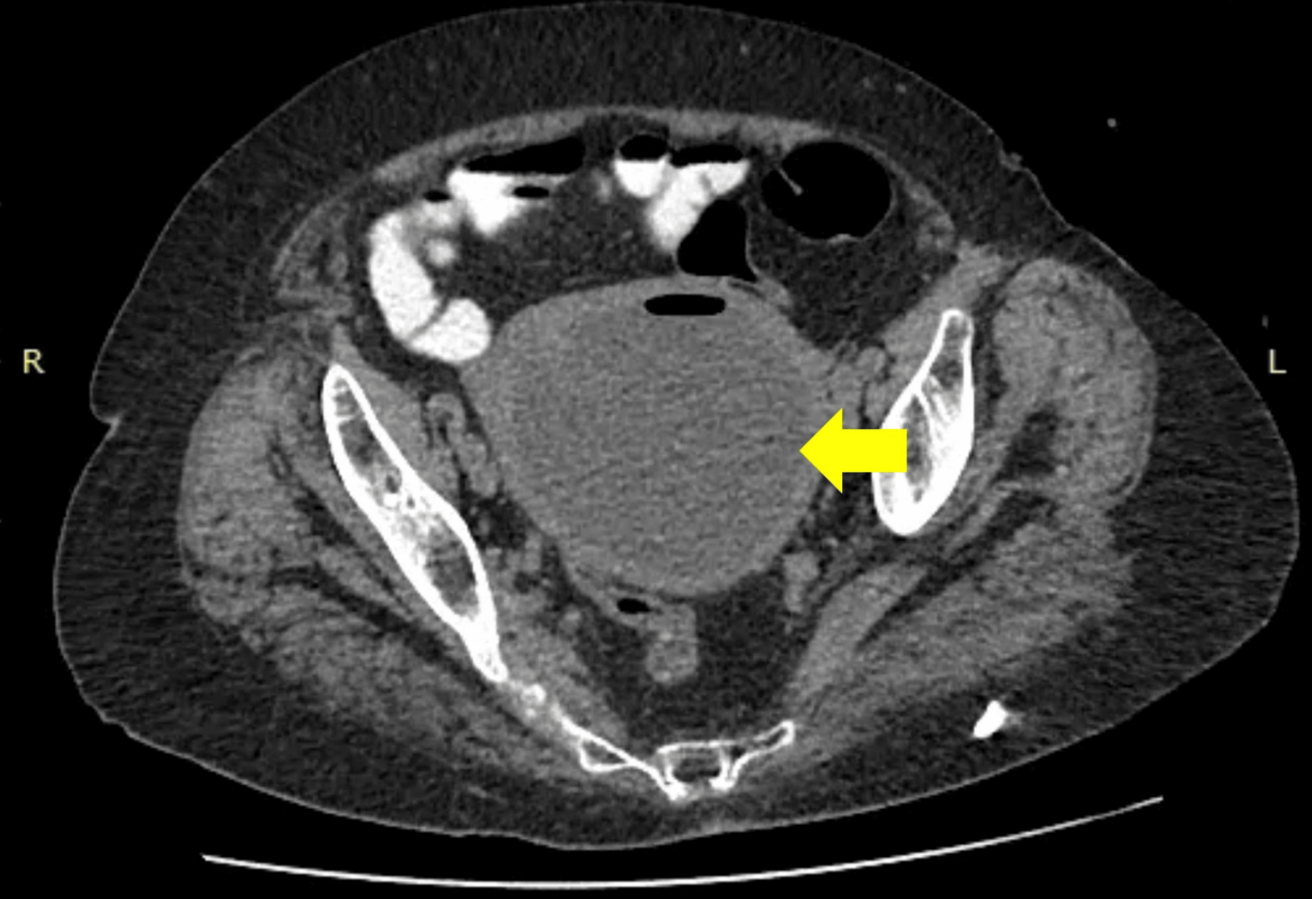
Contrast-enhanced abdominal CT scan showing a distended uterus filled with low-attenuation fluid, consistent with pyometra (yellow arrow) No signs of uterine rupture or free intraperitoneal air or fluid were identified, supporting a diagnosis of unperforated pyometra.
